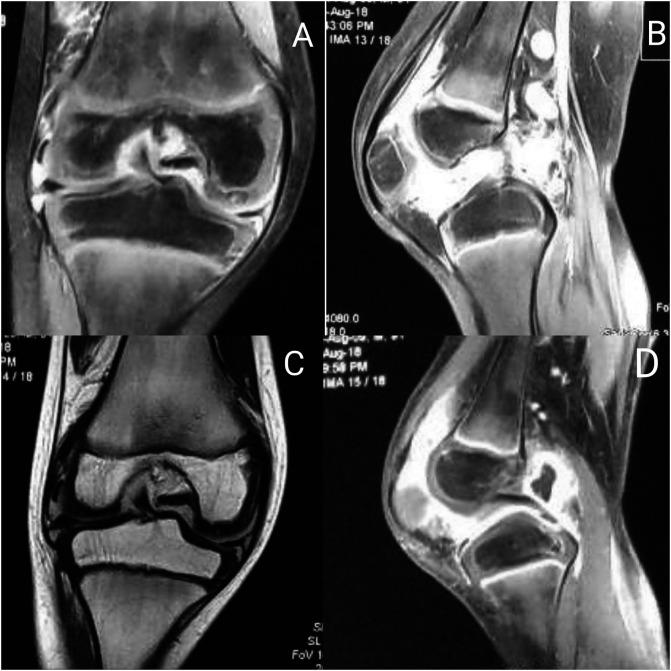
Figure showing MRI sections of a patient with infective pathology (A – coronal STIR, B – sagittal STIR, C – coronal T1, D – sagittal STIR images showing moderate effusion with peripherally enhanced intensities in popliteal fossa).
